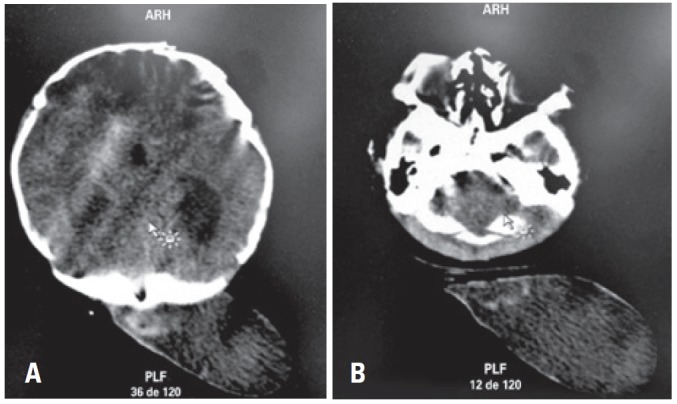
Cranial computed tomography with occipital subcutaneous cystic formation to the left with licorice attenuation of content associated with occipital bone micropuncture in the midline and cortex isodense mass, therefore, suggesting encephalomeningocele
